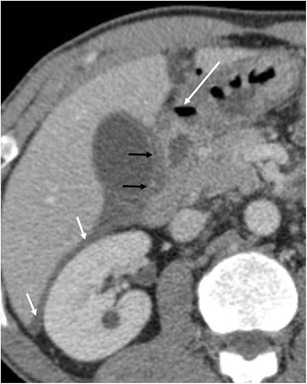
A 48-year-old man who presented with abdominal pain caused by a perforated duodenal ulcer. Axial contrast-enhanced CT shows an irregular air-fluid level (large arrow) adjacent to the duodenal bulb. A leakage of free fluid into the posterior subhepatic space (white arrows) through hepatoduodenal ligament (black arrows) is observed
